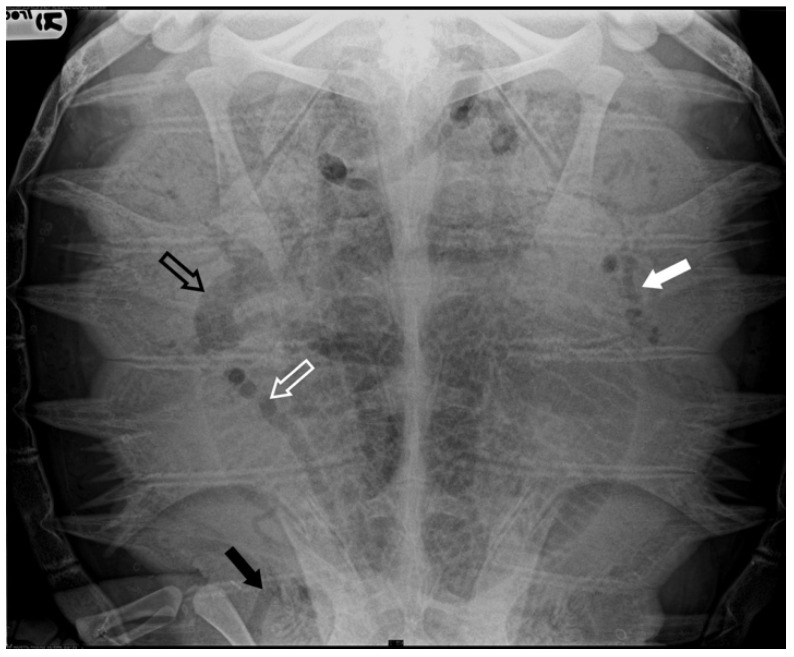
X-ray examination in DV projection of a subject diagnosed with moderate severity GE reveals the presence of gas in various vessels, including the gastric vessels (white arrow), inferior mesenteric artery (white empty arrow), iliac vessels (black arrow), and duodenal vein (black empty arrow). The present gas overlaps the lungs’ cranial area, reducing the visualization of lung volume.
